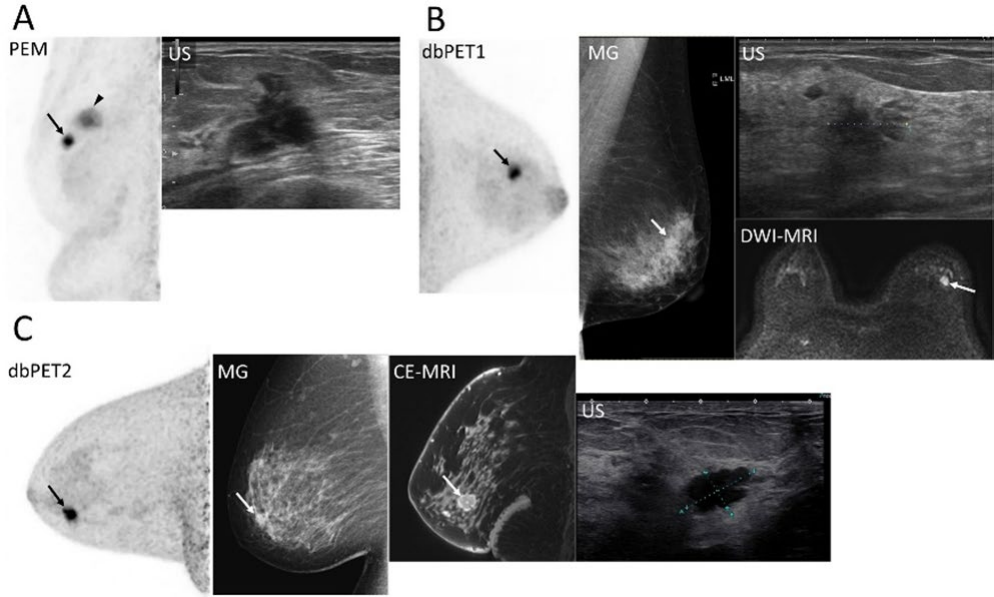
Representative breast-specific PET images for a mass uptake closer to the nipple and inter-modality differences.The primary breast cancer lesions are indicated by arrows. All PET images are displayed using an SUV scale ranging from 0 to 5. (a) A 71-year-old woman with invasive ductal carcinoma (IDC, luminal A): PEM and ultrasonography. A DCIS is also shown by an arrowhead. (b) A 61-year-old woman with IDC (luminal B): dbPET1, mammography (MLO), ultrasonography, and non-contrast MRI (DWI). (c) A 50-year-old woman with IDC (triple negative): dbPET2, mammography (MLO), contrast-enhanced MRI, and ultrasonography. Abbreviations: PET, positron emission tomography; PEM, positron emission mammography; US, ultrasonography; IDC, invasive ductal carcinoma; DCIS, ductal carcinoma in situ; dbPET, dedicated breast PET; MG, mammography; MLO, mediolateral oblique; DWI, diffusion-weighted imaging; CE, contrast-enhanced
